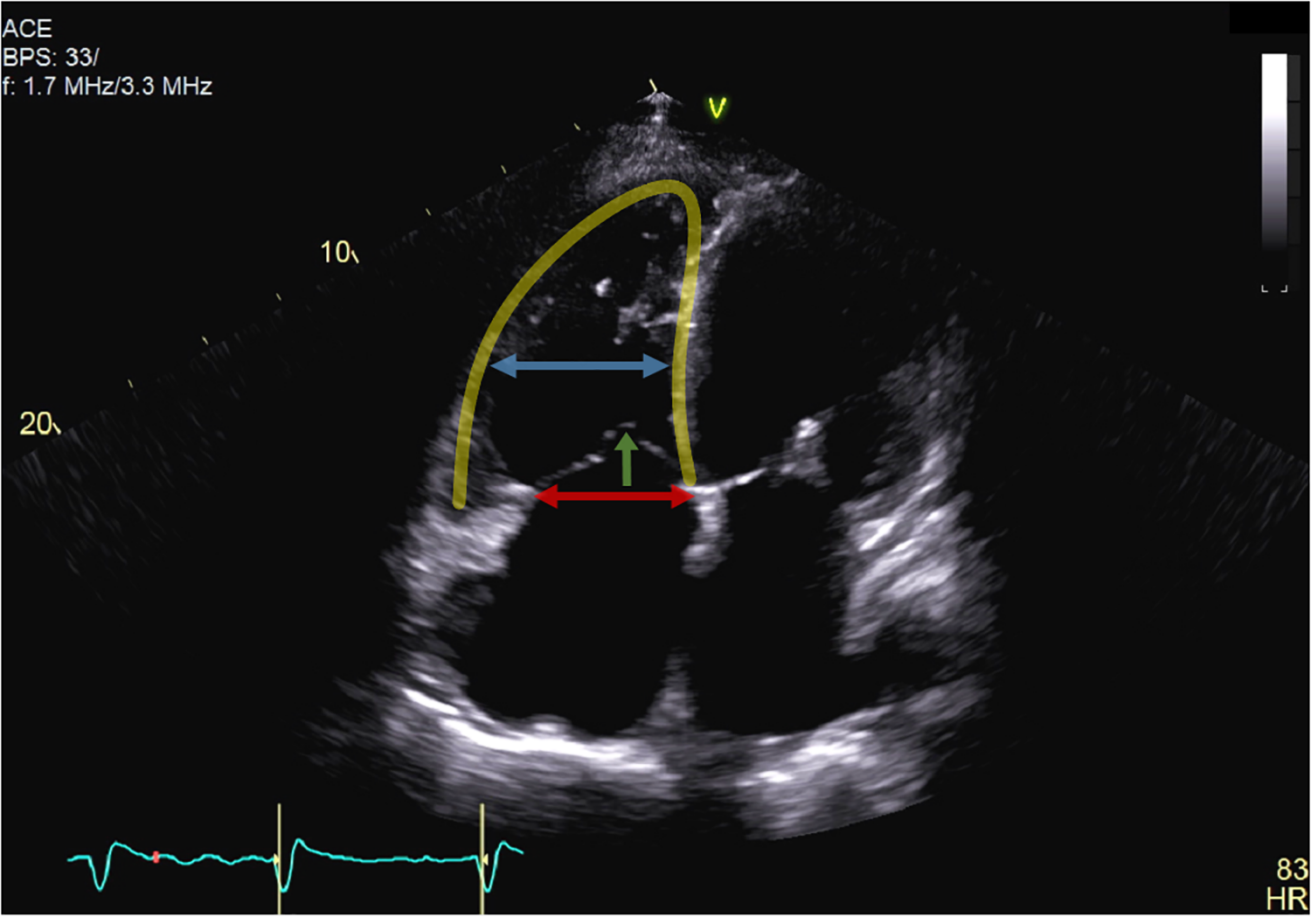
Apical four-chamber view of a patient with ventricular functional tricuspid regurgitation. Tricuspid regurgitation is mainly driven by severe leaflet tethering (green arrow) due to right ventricular dilatation (yellow line) and remodelling. The mid-right ventricular dilatation (blue arrow) was therefore more pronounced than tricuspid annulus dilatation (red arrow), and the line of coaptation was below the tricuspid annulus (>10 mm tenting height).
